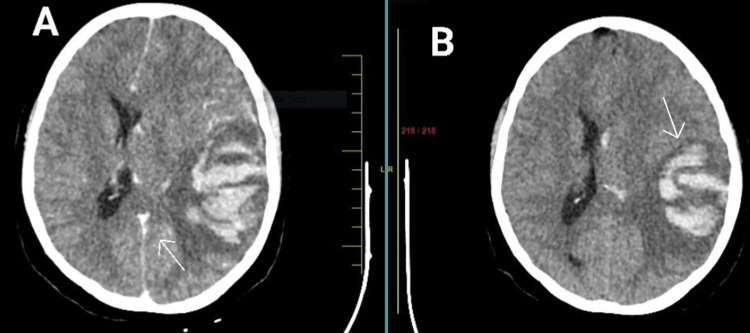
(A) CT brain scan with contrast; arrow indicates the sigmoid sinus thrombosis. (B) Non-contrast CT brain scan; arrow indicates the venous hemorrhagic infarct. Ventricular bleed and midline shift also seen.
